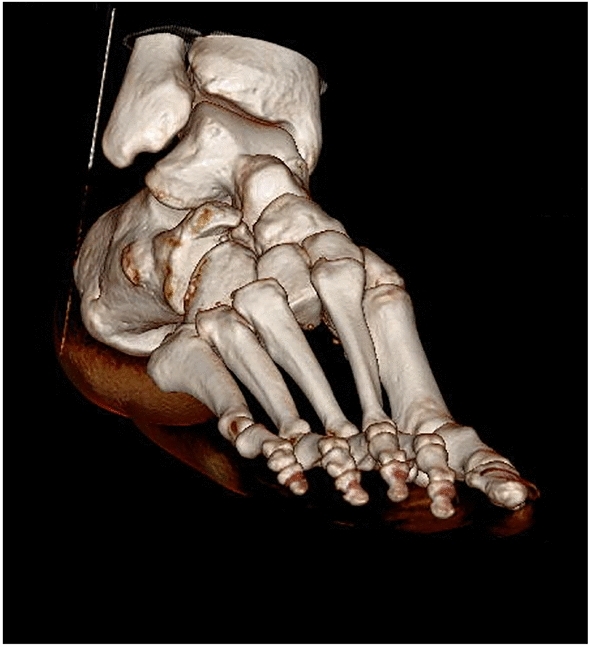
CT three-dimensional reconstructed coronal image demonstrating tarsel-metatarsal dislocation of the second, third, fourth and fifth metatarsals
